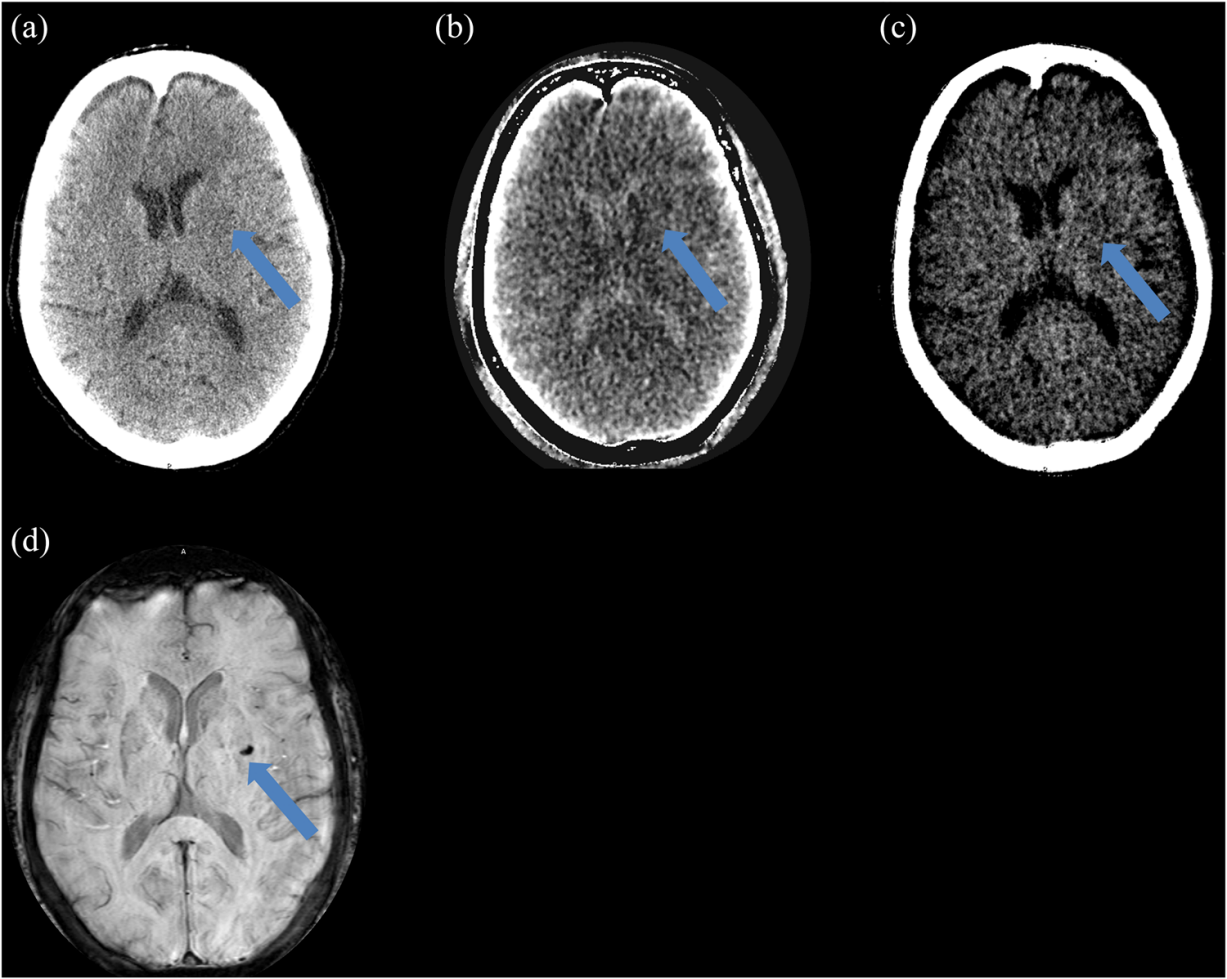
Example of a class 1 hemorrhage disagreement. Male 60 years old with a hemorrhage (denoted by arrows) after tissue plasminogen activator (tPA) administration. Magnetic resonance imaging (MRI) was obtained approximately 18 h after dual energy computed tomography (DECT). (a) 66 keV image is negative due to a lack of hyper density by the area indicated by the arrow, (b) Iodine map is negative for contrast extravasation due to a lack of hyper density by the area indicated by the arrow (c) Virtual non‐contrast image is negative for bleeds due to a lack of hyper density by the area indicated by the arrow, (d) MRI susceptibility weighted imaging (SWI) shows class 1 hemorrhage as a hypodense structure by the arrow.
